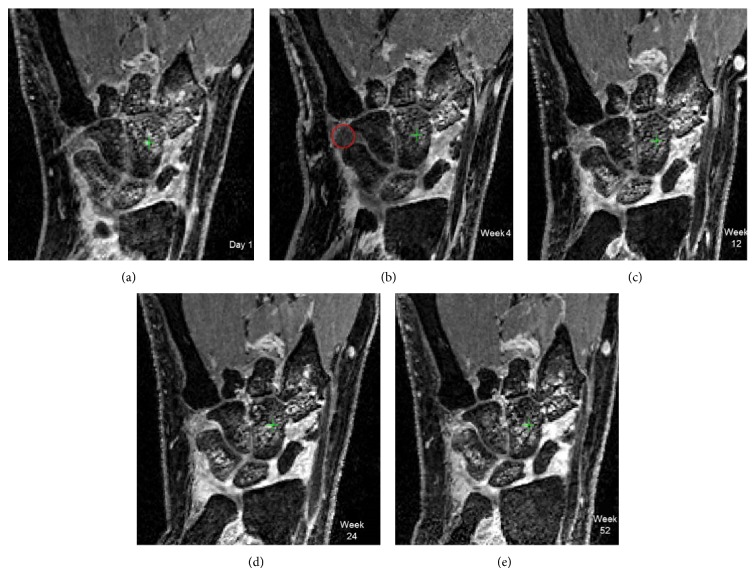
Image shows wrist MRI disease activity at (a) day 1, (b) week 4, (c) week 12, (d) week 24, and (e) week 52 in patient with early disease (disease duration 5 months at baseline). Over the year, there is large amount of BME (RAMRIS scores: 28, 26, 27, 22.5, and 23 at day 1, week 4, week 12, week 24, and week 52). The patient also had rapid loss in RC-BMD at week 12. There is also marked synovitis in the wrist joint with transient improvement at week 4 distal to triquetrum (red circle).
